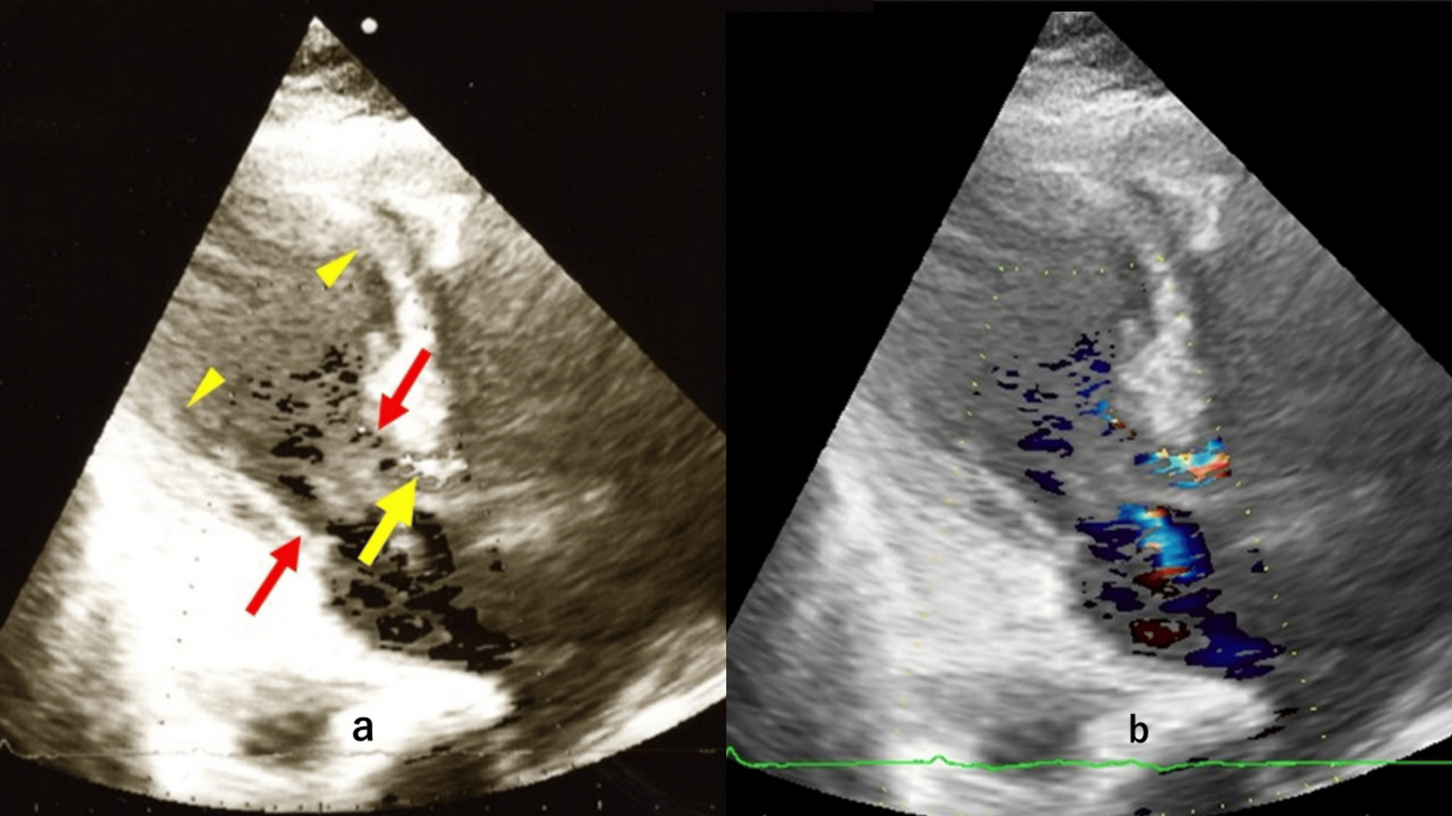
EC in ICU after CAG a: During the bedside echocardiography (EC) in the intensive care unit (ICU) at this time, the left ventricle showed akinesis from the apex to the interventricular septum and posterior wall (yellow wedges), with only the base exhibiting excessive contraction (red arrows). Echocardiographic Doppler measurement revealed a pressure gradient reaching 160 mmHg (yellow arrow). b: Furthermore, there was evidence of the anterior movement of the mitral valve anterior leaflet during systole, and severe mitral regurgitation was also present. CAG: coronary angiography
